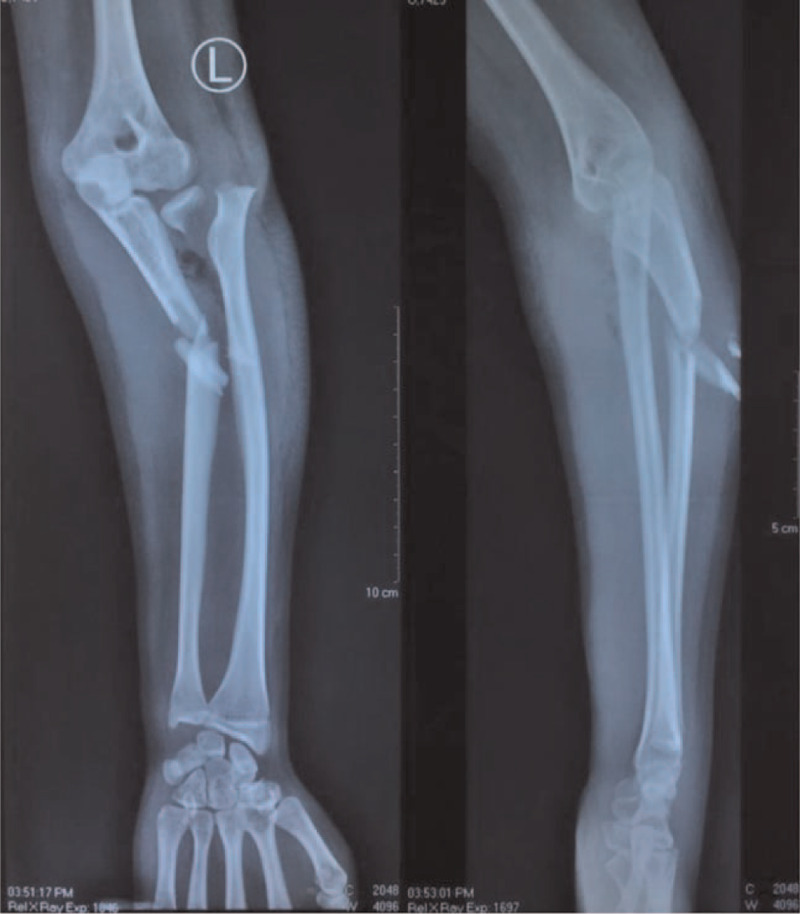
The plain radiograph of the patient's left forearm, anteroposterior (AP) and lateral (L) view.
